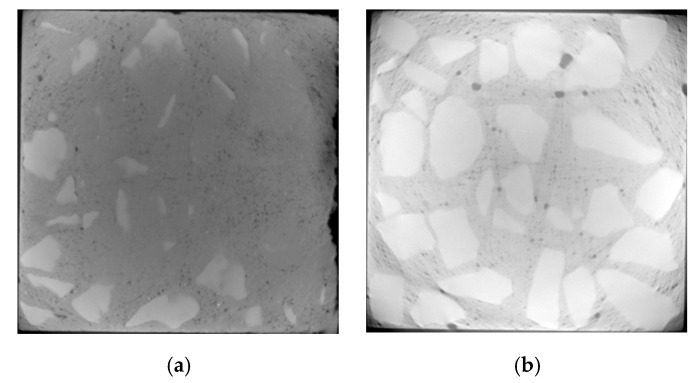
CT images of D4 before sulfate attack (no cracks): (a) surface layer; (b) inner layer.
